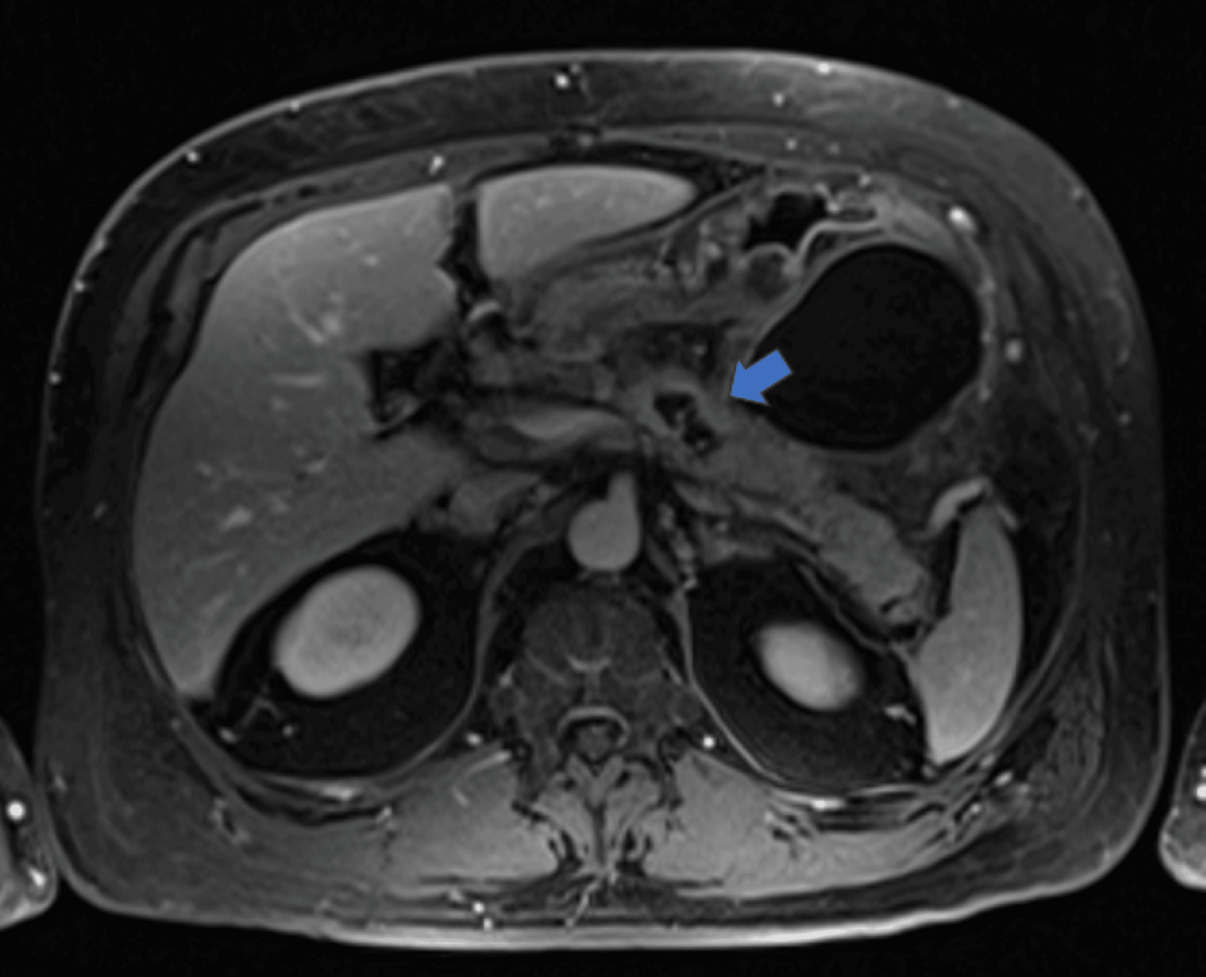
Magnetic resonance imaging-cholangiopancreatography obtained three weeks after Figure 1, with the blue arrow indicating necrosis of the pancreas.
